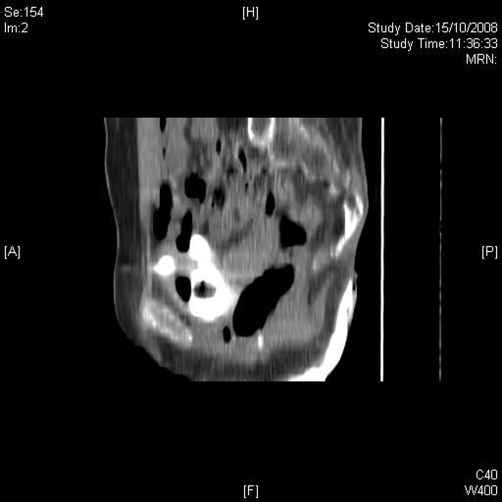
Sagittal reconstruction of CT cystography, performed on 15 October 2008, shows hourglass bladder with narrow waist.
